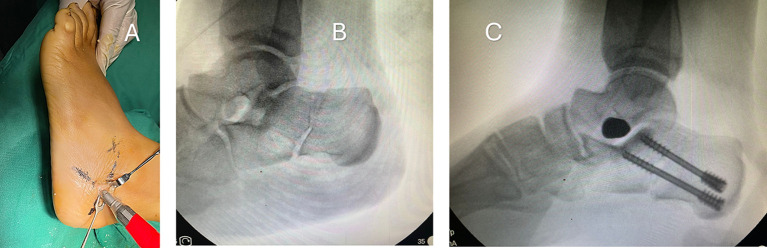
A: Intraoperative photograph of MIS MDCO using low-speed, high-torque burr. B: Intraoperative X-ray after completion of MDCO. C: MDCO after fixation with 2 screws.
